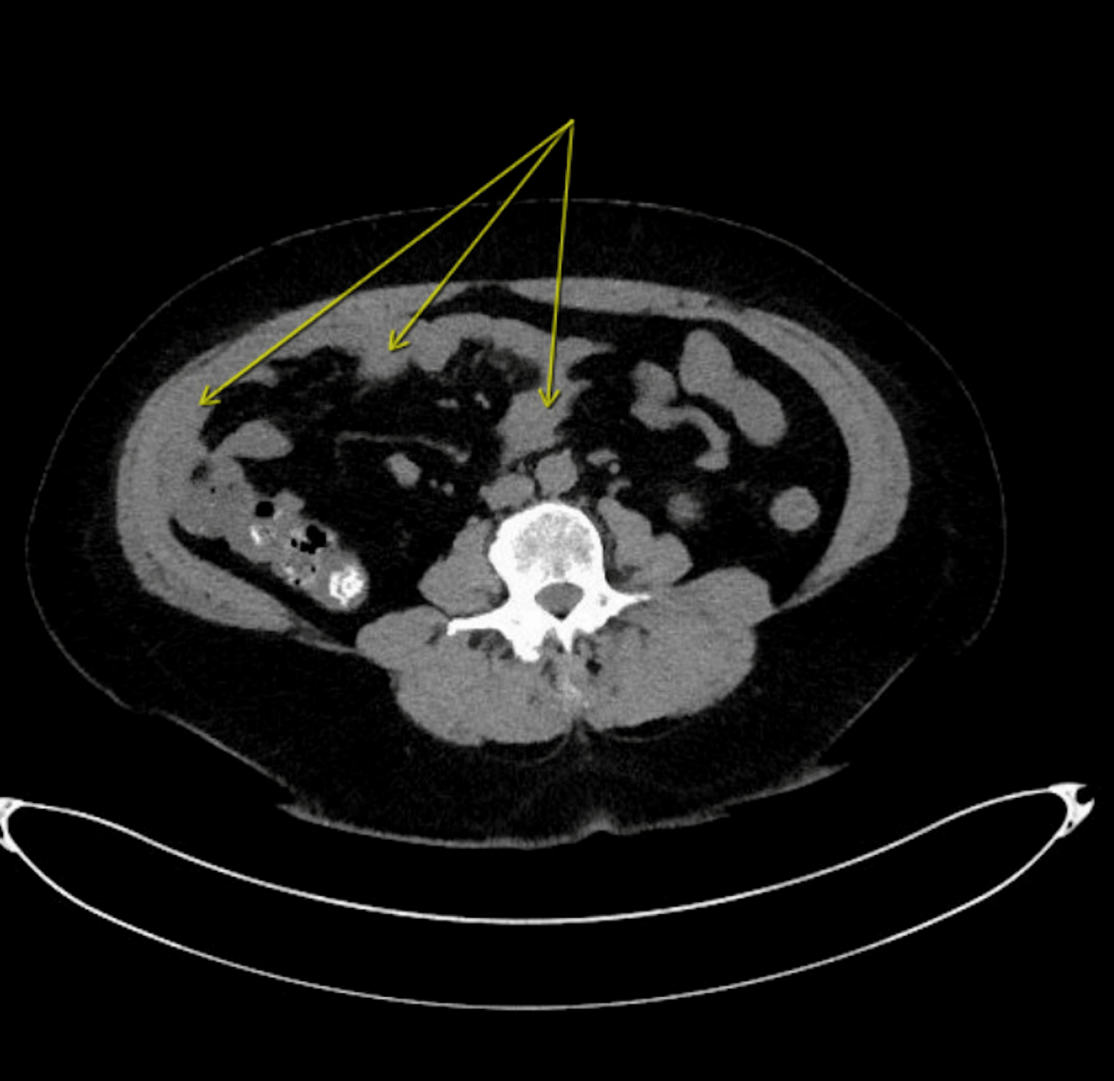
CT of the abdomen and pelvis without contrast Imaging demonstrates wall thickening of multiple small bowel loops (arrows) suggestive of enterocolitis.
